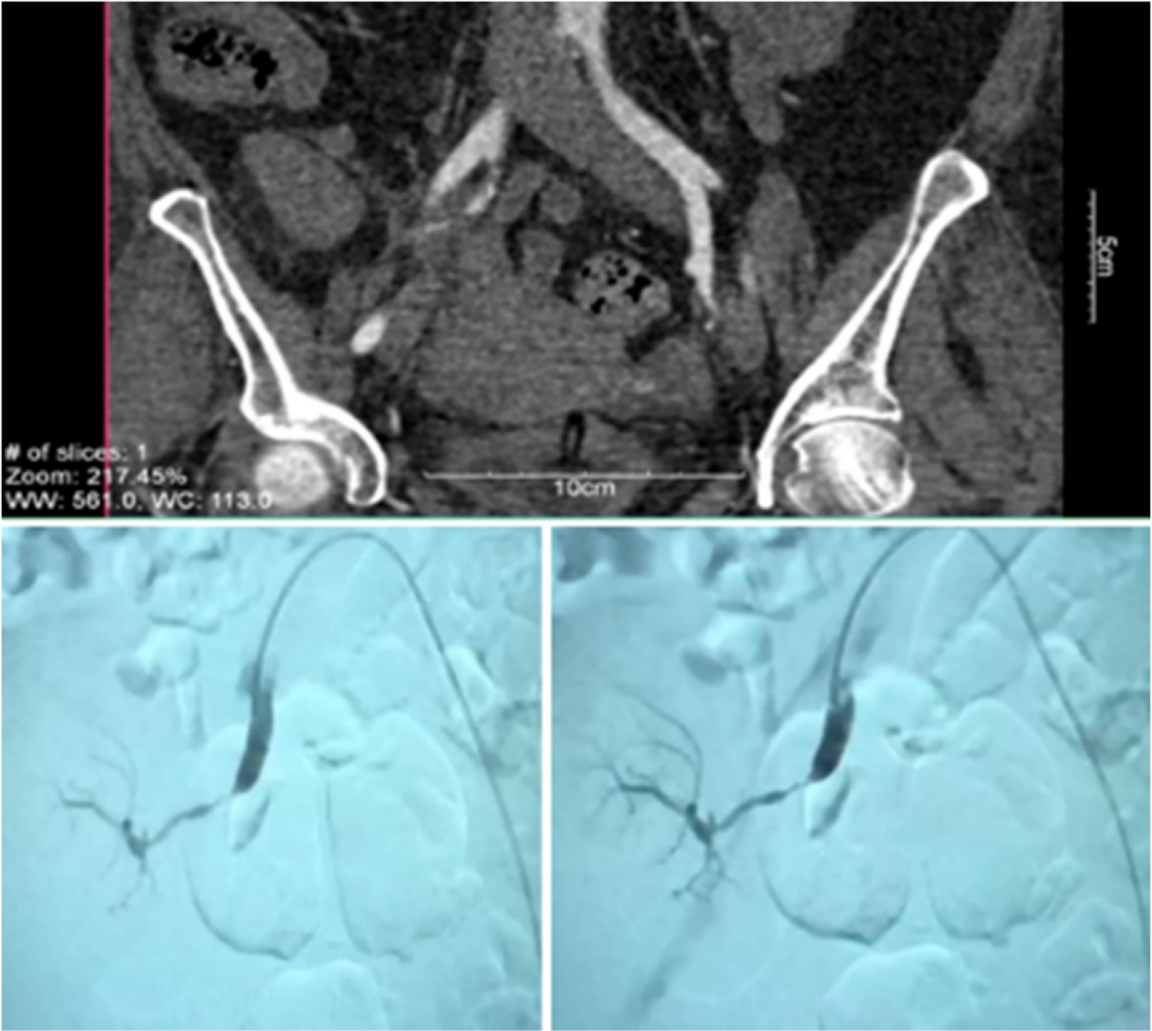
CT angiography of abdomen and pelvis and renal angiography showing an 80% or greater stenosis of the origin of the transplant renal artery.
